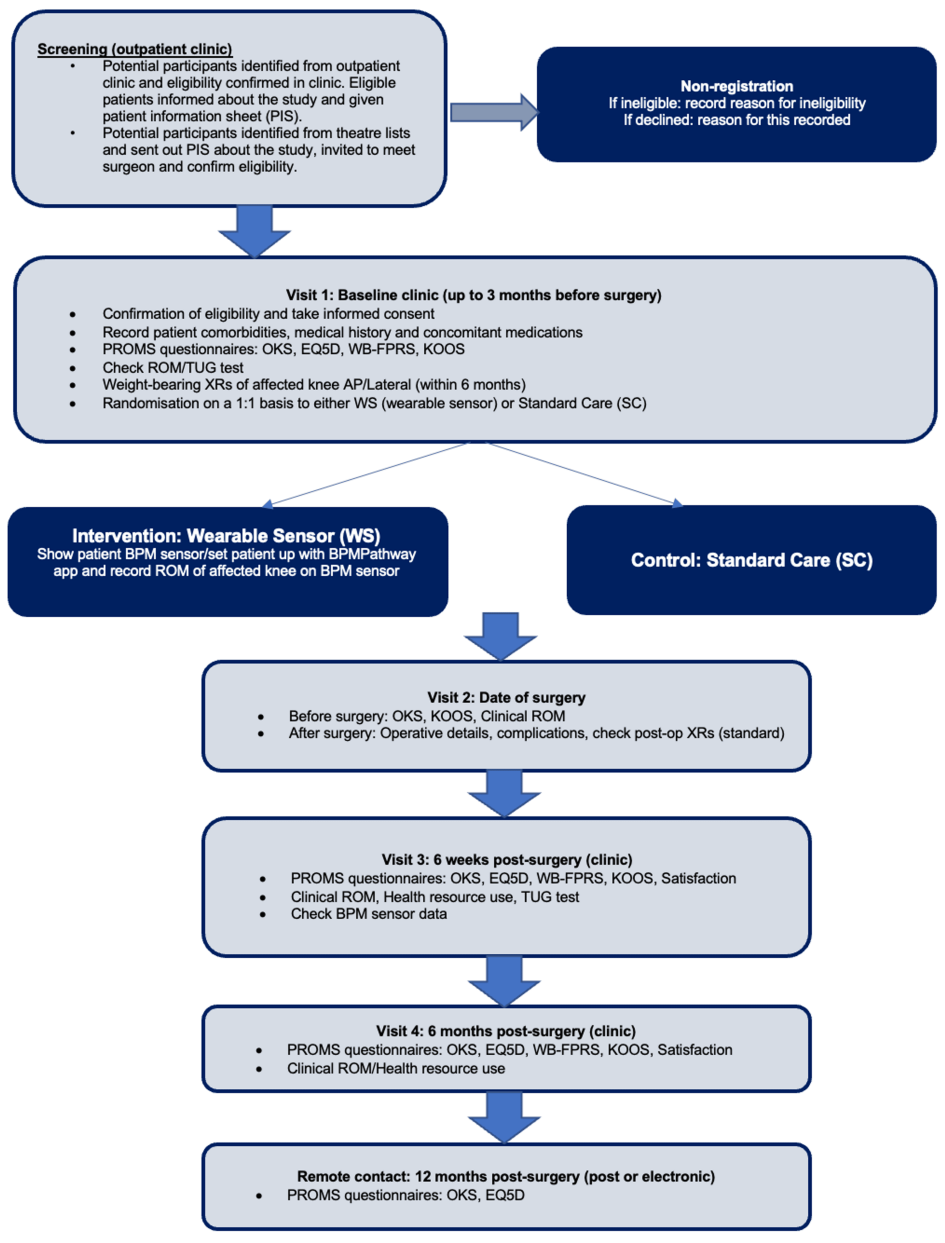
Recruitment and study timeline process OKS: Oxford knee score; EQ5D: EuroQuol-5 dimensions; WB-FPRS: Wong-Baker FACES Pain Rating Scale; KOOS: knee and osteoarthritis outcome score; ROM: range of motion; TUG: timed up and go test; PROMS: patient-reported outcome measures; XR: X-ray; AP: anteroposterior
